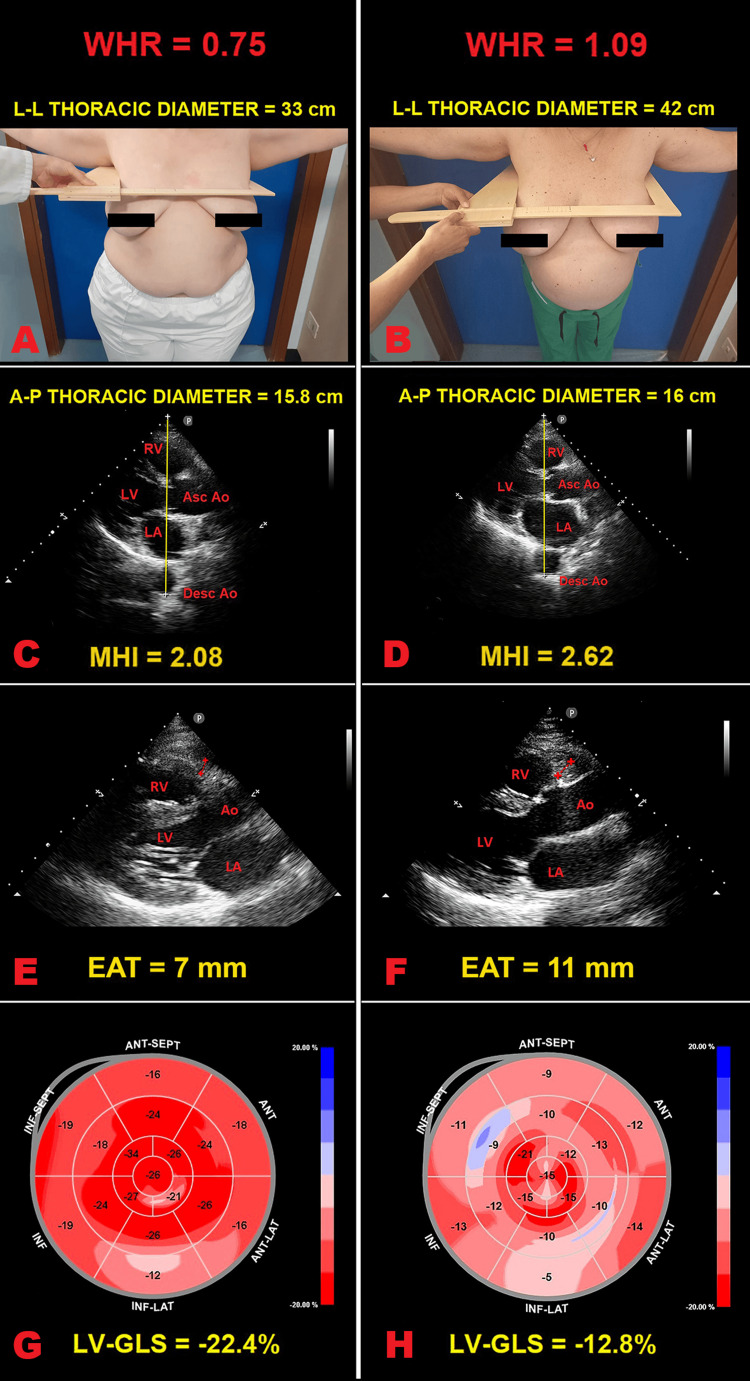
Examples of L-L thoracic diameter (panels A and B), A-P thoracic diameter (panels C and D), EAT (panels E and F), and LV-GLS bull’s eye plot (panels G and H) assessment in healthy women with gynoid obesity and in a healthy woman with android obesity respectively, enrolled in the present study. Panels A and B: The maximum L-L external thoracic diameter, measured with the obese woman in the standing position and with open arms, by using a rigid ruler in centimeters coupled to a level (the measuring device), placed at the distal third of the sternum, in the point of maximum depression of the sternum. Panels C and D: The minimum A-P internal thoracic diameter, obtained with the obese women in left-lateral decubitus position, during the conventional transthoracic echocardiography, by placing a 2.5 mHz transducer near the sternum in the left third or fourth intercostal space, to obtain a parasternal long-axis view, and measuring the distance between the true apex of the sector (the point of entry of ultrasound) and the anterior surface of the vertebral body. The vertebral body is identified by using, as a reference point, the posterior wall of the descending thoracic aorta, visualized behind the left atrium. Panels E and F: End-systolic EAT thickness, measured on the free wall of the right ventricle, from the parasternal long-axis view on standard 2D-TTE. Panels G and H: Examples of LV-GLS bull’s eye plot, assessed by 2D-STE analysis. The healthy woman with “pear-shaped” obesity (WHR = 0.75) showed an uniformly red pattern of the bull’s eye plot, representing a normal range in strain values (Panel G), whereas the healthy woman with android obesity (WHR = 1.09) showed a significant impairment in LV-GLS magnitude (Panel H). 2D, two-dimensional; Ao, aorta; A-P, antero-posterior; Asc, ascending; Desc, descending; EAT, epicardial adipose tissue; GLS, global longitudinal strain; LA, left atrium; L-L, latero-lateral; LV, left ventricle/ventricular; MHI, modified Haller index; RV, right ventricle; STE, speckle tracking echocardiography; TTE, transthoracic echocardiography; WHR, waist-to-hip ratio.
